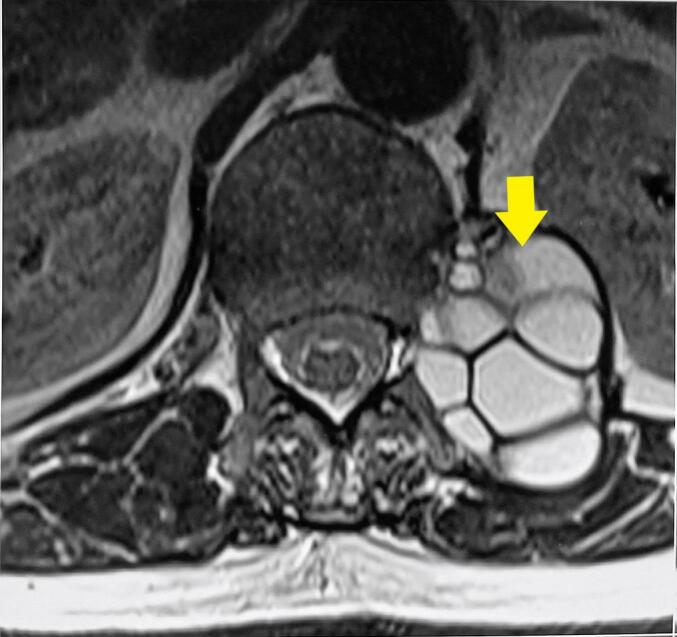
Preoperative thoracolumbar MRI. Axial T2-weighted image revealing the large multiloculated cystic lesion in the left paravertebral region (yellow arrow), with compression and displacement of surrounding structures particularly the left kidney. There is also a close contact the left hemi vertebral body and foramen.
